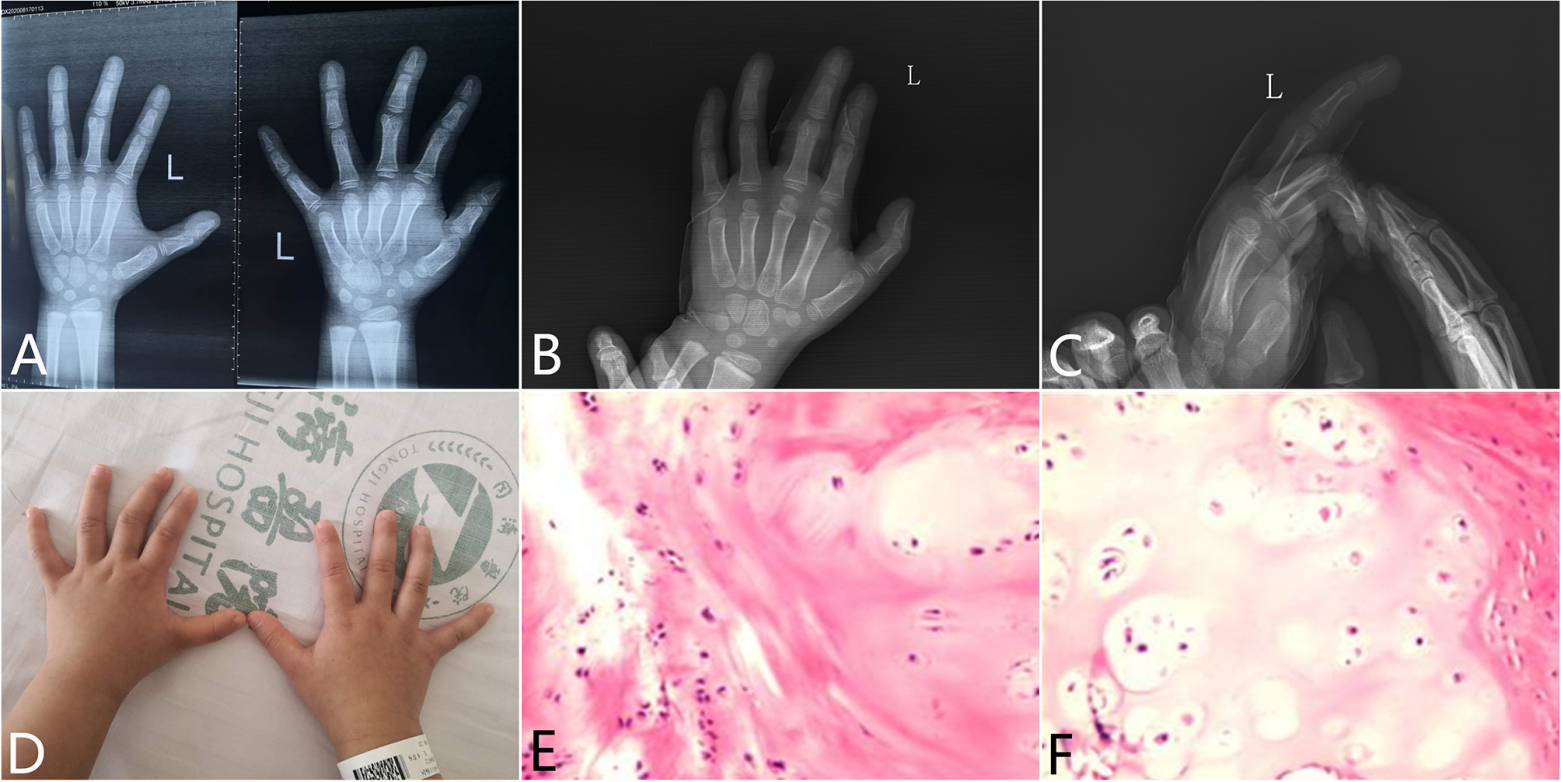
Diagnosis and treatment of clinodactyly deformity in a 4-year-6-month-old girl. X-ray radiography showed a mass protruded into the PIP joint which made the axis declined (A). During the surgery, the abnormal mass was resected without K-wire implantation (B, C). Clinical examination established the diagnosis of clinodactyly deformity of her third finger (D). Pathology examination proved to be osteochondroma (E, F).
